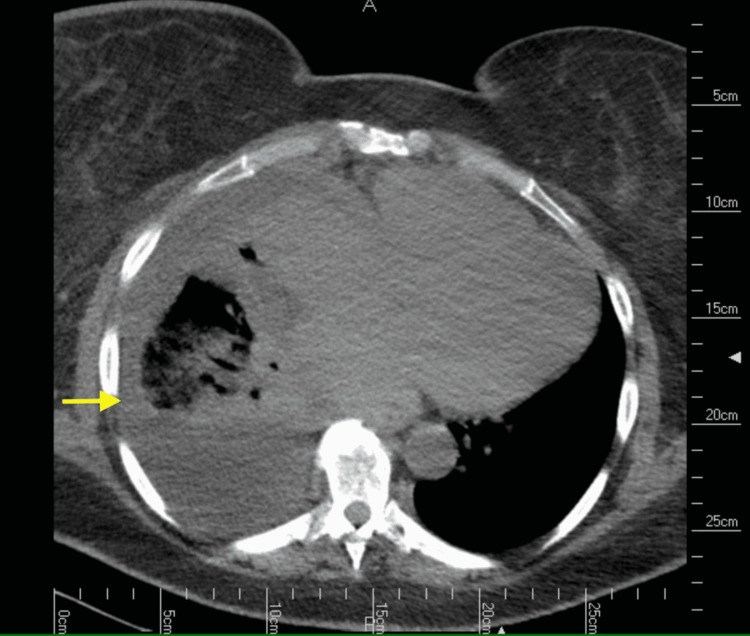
Chest CT scan (axial view) showing a right lower lobe pneumonic consolidation with a moderate-sized pleural effusion (yellow arrow).
